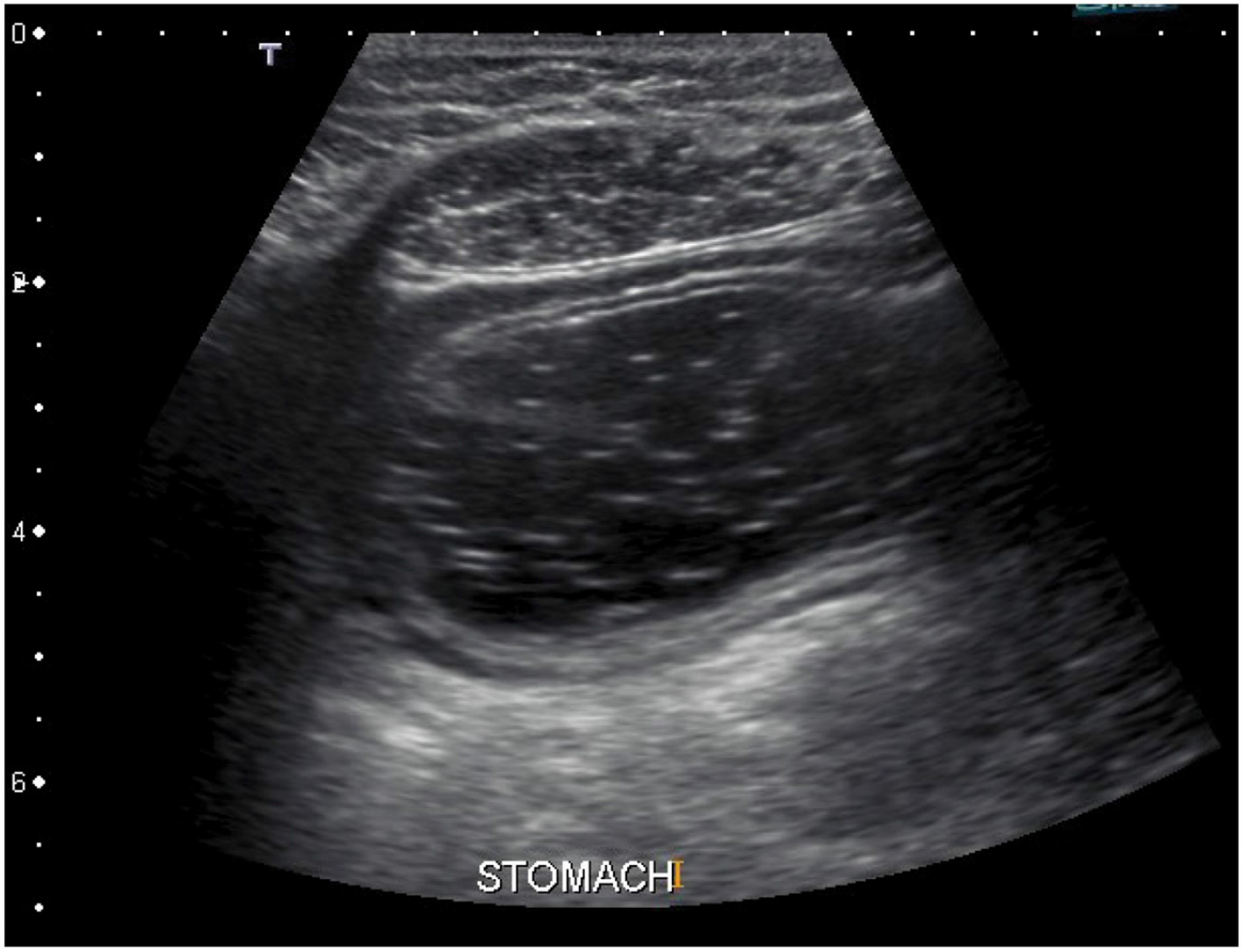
An ultrasound image of the stomach. The stomach is visible as dark area in the middle of the image. The image is taken from transversal plane (57).
